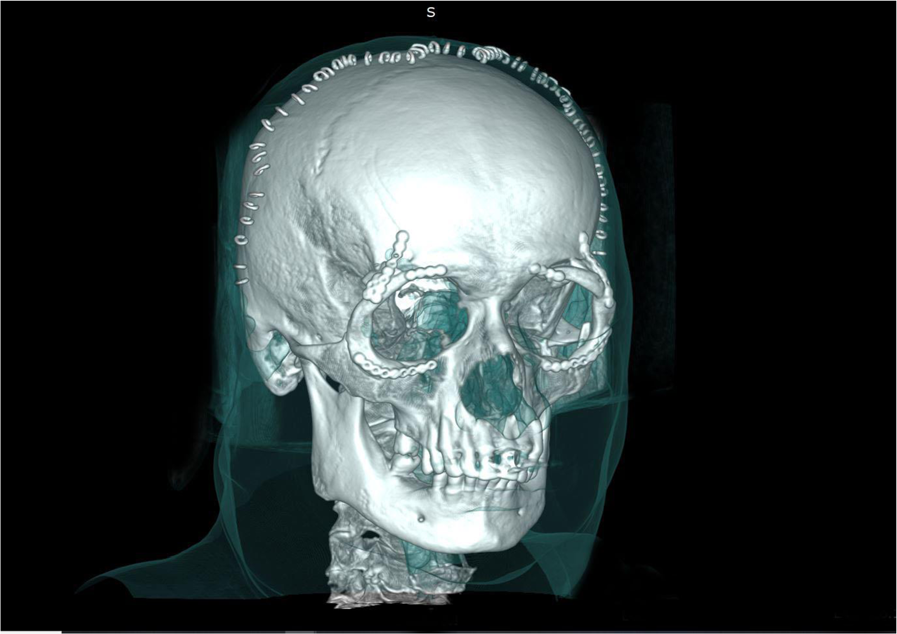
Postoperative CT after 3 wall decompression surgeries with LARA on both sides
